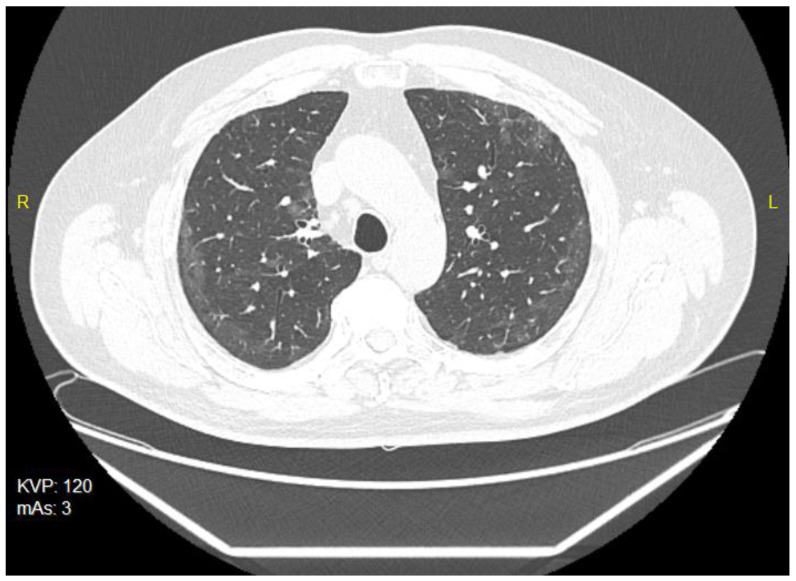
The chest CT scan of 5 February 2021—bilateral ground-glass opacities.
